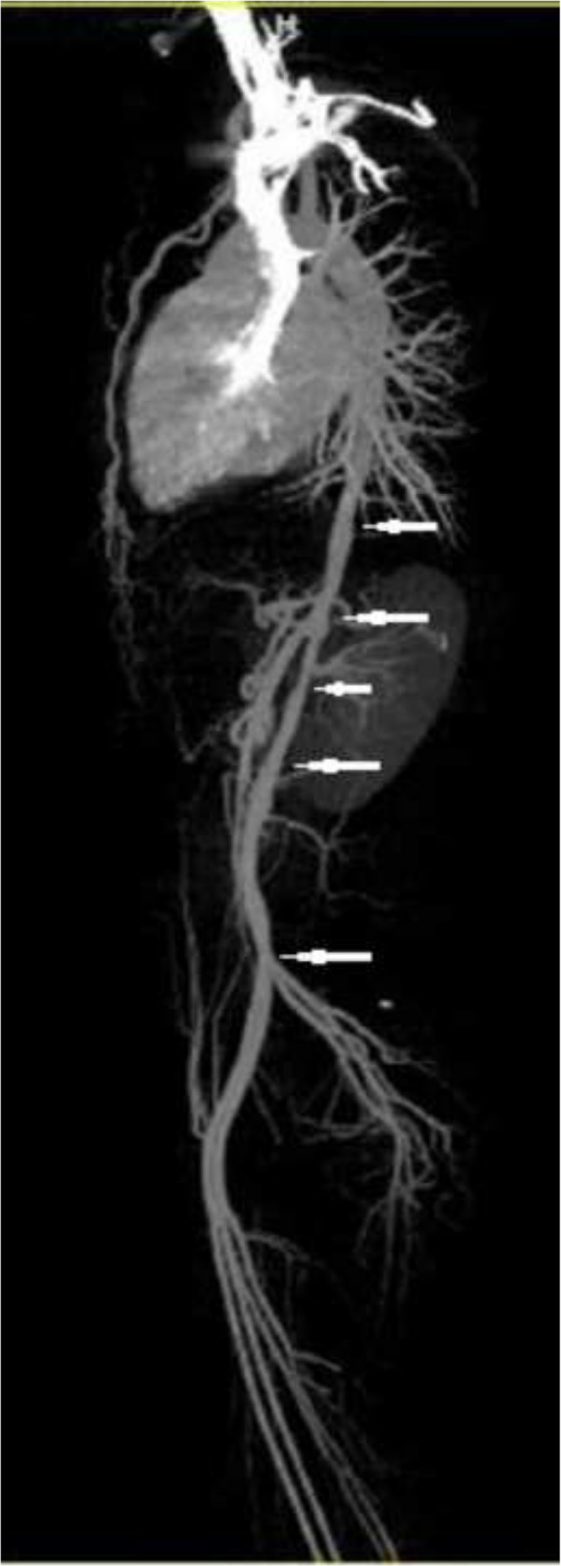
Computed tomography angiography of the thoracic and abdominal aorta showing irregular narrowing with variable degrees of stenosis (*arrows*), tapering and corrugated appearance
